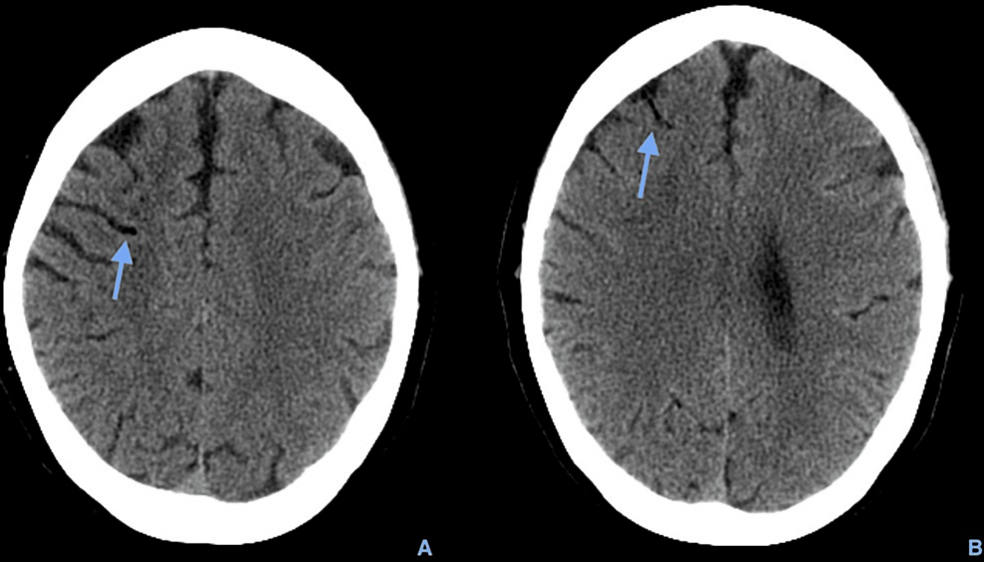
Axial brain CT scan showing serpiginous hypodensity in the high right anterior frontal high convexity (blue arrow on A and B), compatible with air in the sulci, with no ischemic lesion objectified
